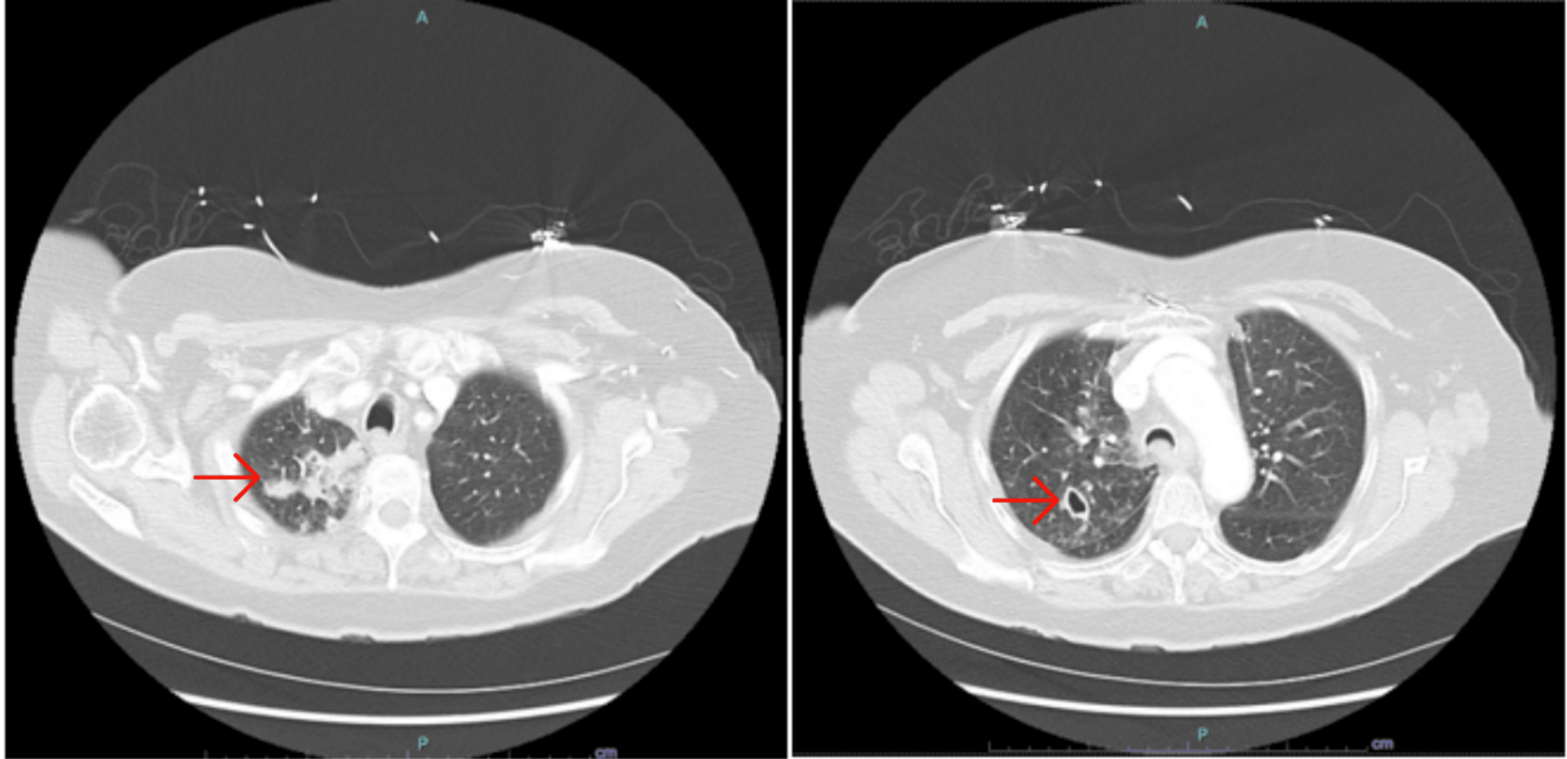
CT chest performed inpatient demonstrated a new thin-walled cavitary lesion in RUL and consolidations in RML and apex. RUL: right upper lobe; RML: right middle lobe
